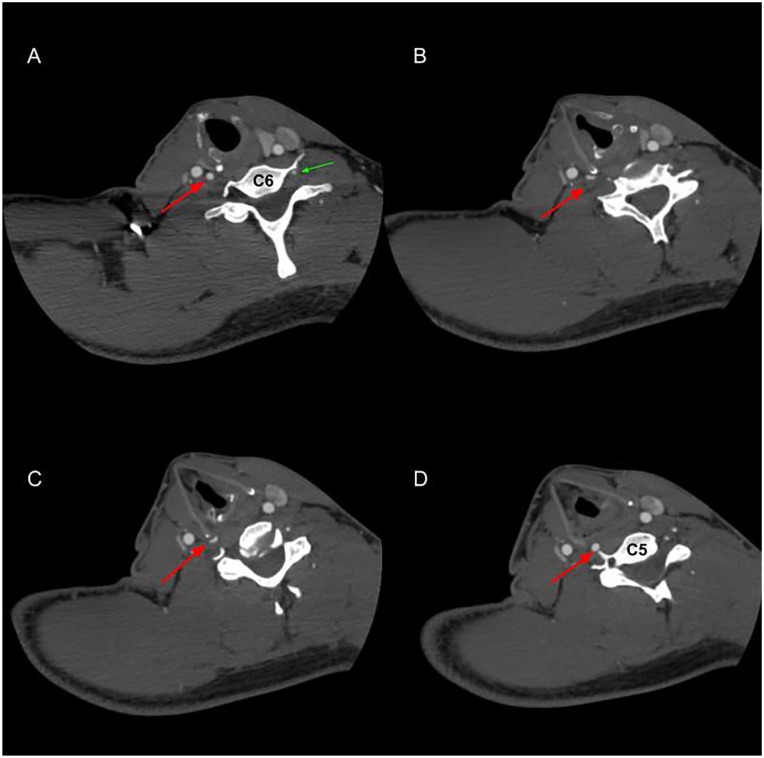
Computed tomography (CT)-scan of the neck during maximal ipsilateral
